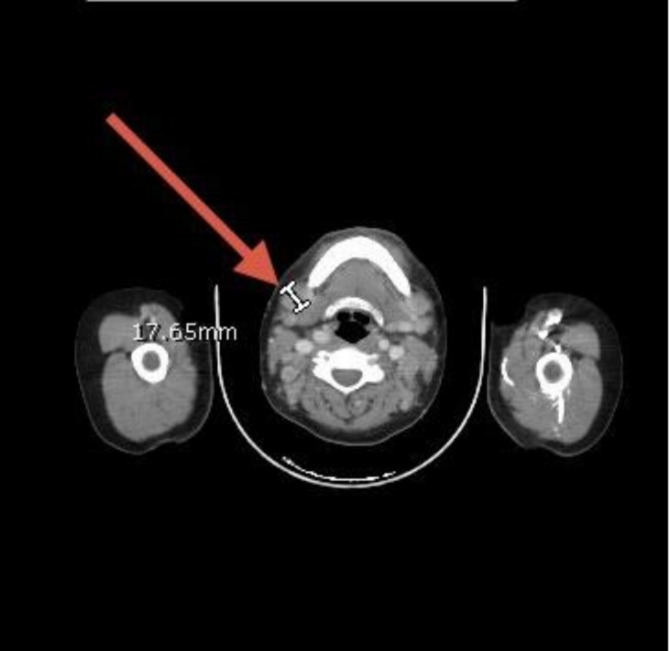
Cervical lymphadenopathy. Contrast‐enhanced CT of the neck showing multiple enlarged bilateral cervical lymph nodes across all nodal levels, consistent with pathological lymphadenopathy.
